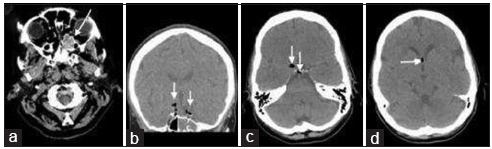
A 62-year-old woman presenting with headache and vertigo found to have a mucopyocele and pneumocephalus. (a) Non-contrast-enhanced axial CT image of the head demonstrates hyperattenuation within an expanded left posterior ethmoid air cell (arrow), initially considered a chronic mucocele with inspissated secretions, but later found to be a mucopyocele intraoperatively. (b) Coronal CT reformation and (c,d) axial non-contrast CT images of the head demonstrate foci of pneumocephalus (arrows) in the parasellar region, suprasellar cistern, and cavum septum pellucidum, suspicious for an intracranial extension.
